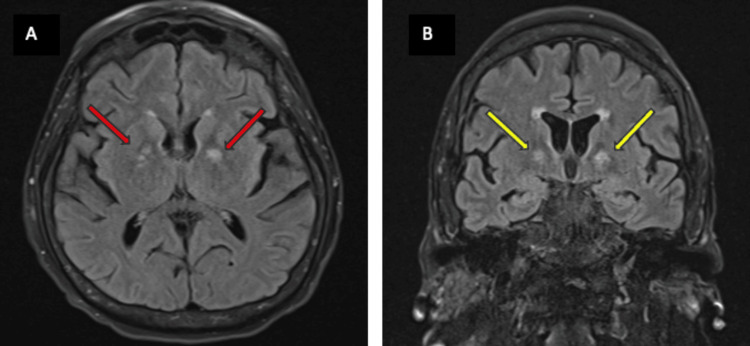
Axial MRI FLAIR (yellow arrows) (A) and coronal MRI FLAIR (yellow arrows) (B) demonstrating corresponding hyperintensities MRI: magnetic resonance imaging; FLAIR: fluid-attenuated inversion recovery
